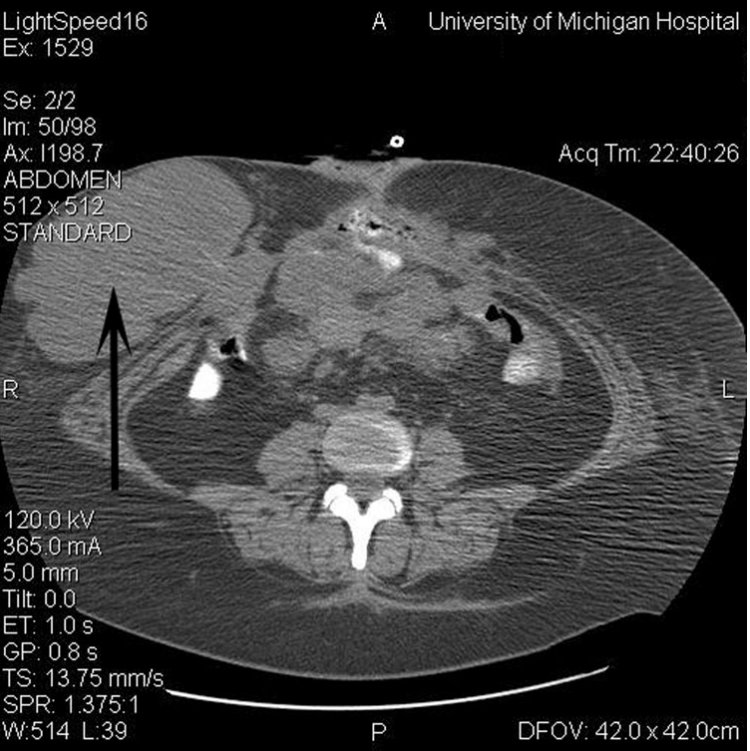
Computed tomogram of the abdomen prior to initiation of cytotoxic chemotherapy demonstrating right-sided abdominal wall soft tissue mass (16 × 9 cm) (arrow), and mesenteric soft tissue mass (7 × 5 cm).
